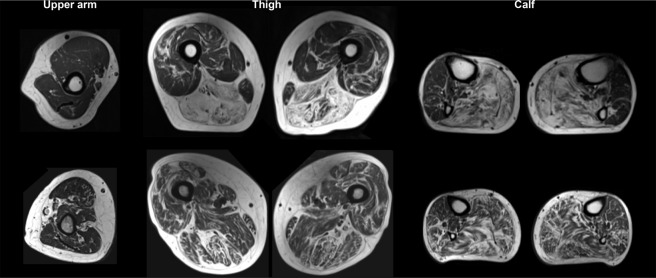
MR images of upper arm, thigh and calf muscles in one patient with spinobulbar muscular atrophy T1-weighted MR images of the upper arm, thigh and calf in two patients with spinobulbar muscular atrophy.
